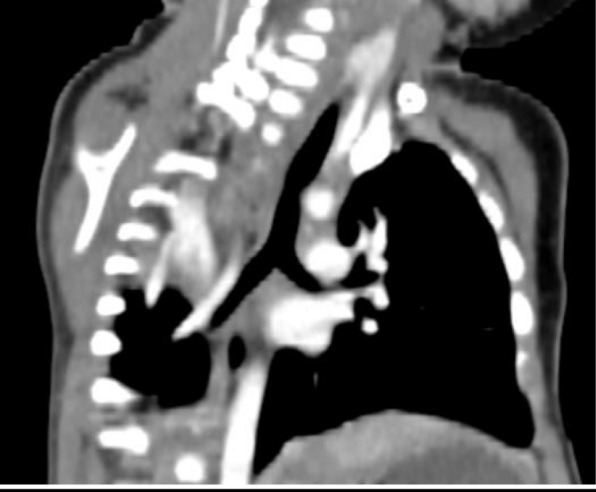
Coronal CT image of one year old boy in mediastinal window shows partial absent right lung (upper and middle) with existence of right lower lobe with its bronchus. The heart locates in the right upper zone
